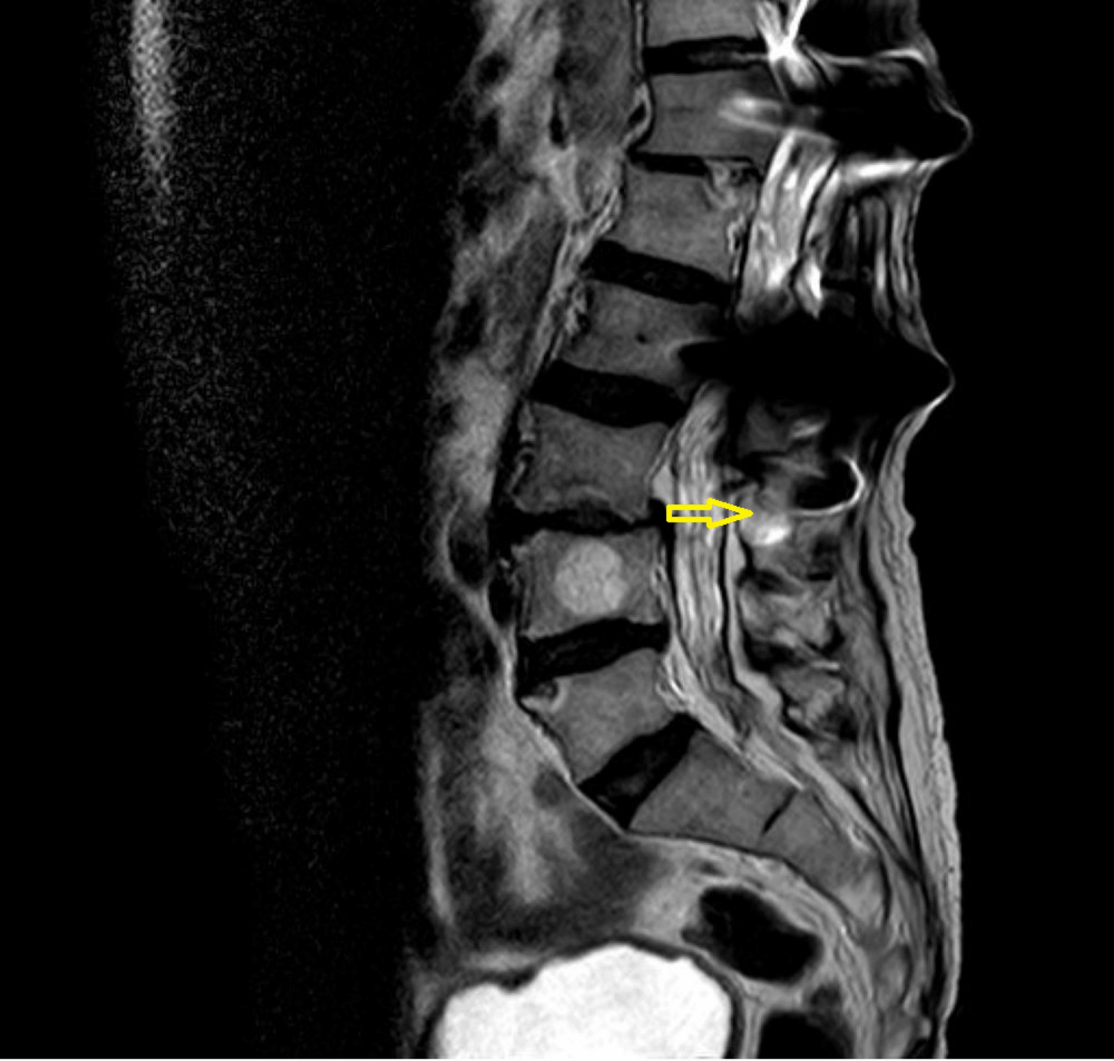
MRI is favorable in depicting arachnoiditis. Coronal T2-weighted image shows diffuse abnormal circumferential thickening of thecal sac with blooming artifact, concordant with dural calcifications seen on CT image. (Image published under Creative Commons License; image credits: case courtesy of Noriza Zainol Abidin, Radiopaedia.org, rID: 51394)
